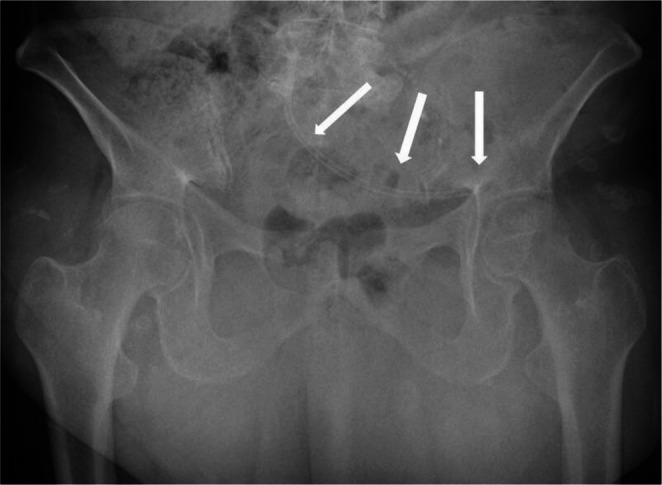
An anteroposterior hip radiograph. A curvilinear biliary stent is seen extending from the mid-pelvis into the left lower quadrant, passing over the iliopectineal and ilioischial lines, with the distal lateral end of the stent terminating at the left acetabular roof (arrows)
